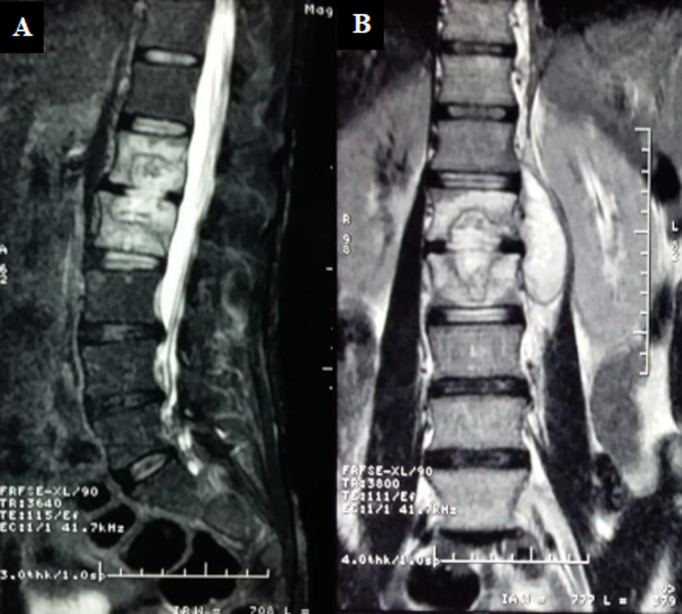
sequential T2-weighted magnetic resonance images of the lumbar spine; A) sagittal; B) coronal views, showing spondylitis L1L2 with collections of the vertebral bodies and in the left psoas
